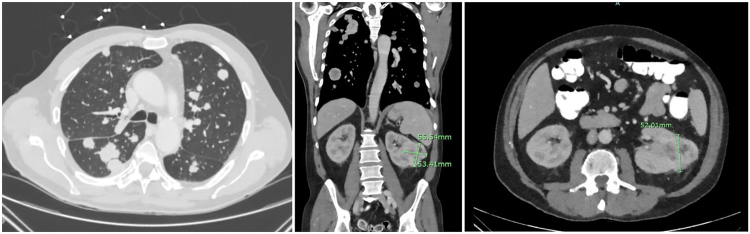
Contrast-enhanced CT of the Chest, Abdomen, and Pelvis. (A) CT imaging revealing multiple pulmonary nodules. (B) CT showing a lobulated, exophytic heterogeneous enhancing soft tissue mass in the left kidney measuring approximately 5.5 × 5.2 × 5.3 cm. (C) CT demonstrating similar renal mass findings with concerns for portal vein thrombus.
